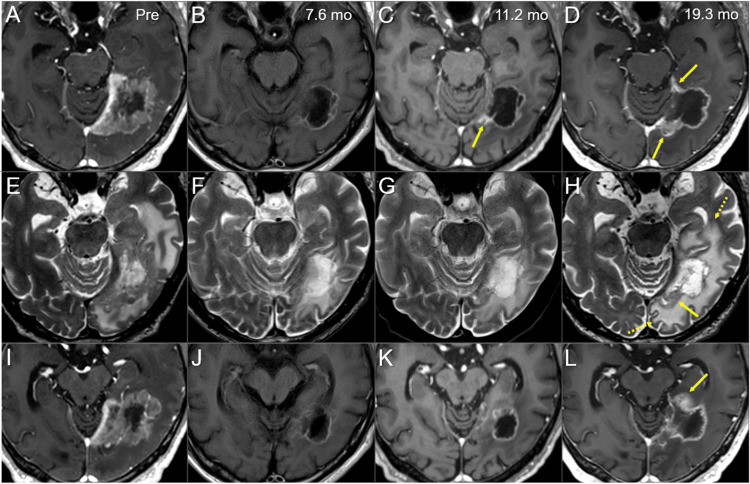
Magnetic resonance images before and after the radiosurgery followed by whole-brain radiotherapy The images show (A-D, I-L) axial CE T1-WIs, (E-H) axial T2-WIs; (A, E, I) before the SRS initiation, (B, F, J) at 7.6 mo after the SRS initiation, (C, G, K) at 11.2 mo and (D, H, L) at 19.3 mo. (A-L) These images are shown at the same magnification and coordinates under co-registration and fusion based on the pre-SRS images (A-H, the same cross-section; I-L, different cross-section). (B, F, J) The solid component, including the infiltrated region into the tentorium, almost completely regressed, leaving only slightly enhancing effects at the boundary. The perilesional edema decreased markedly, and the mass effects almost disappeared. (C, G, K) Part of the enhancing effects near the tentorial incisura slightly increased (arrow in C), which was deemed as adverse radiation effects at that time. (D, H, L) The enhancing lesions further enlarged (arrows in D, L), along with the corresponding mass on a T2-WI (arrow in H) and the increased perilesional edema (dashed arrows in H). CE: contrast-enhanced; WI: weighted image; SRS: stereotactic radiosurgery; mo: months
